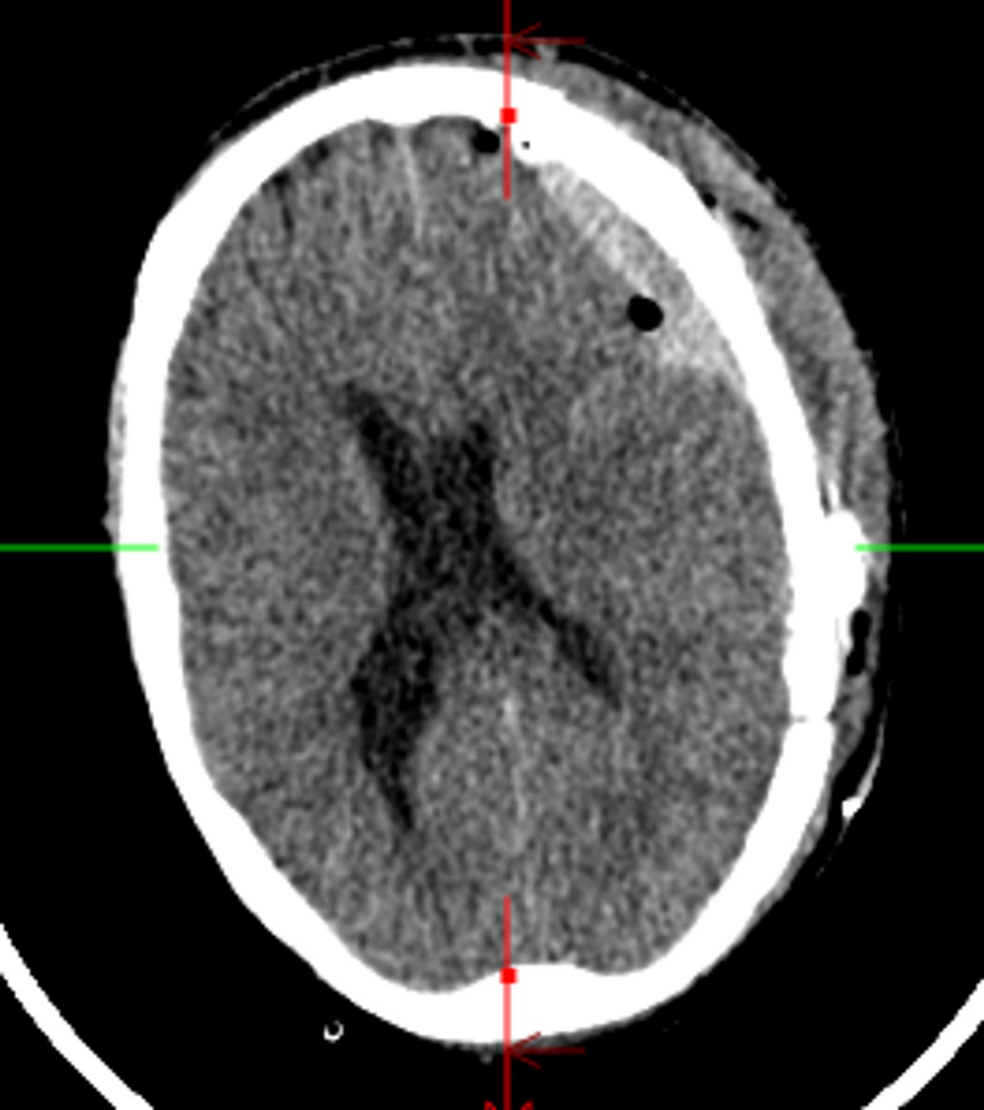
Noncontrast axial head computed tomography (CT) after decline in examination showing SDH without interval worsening. SDH = subdural hematoma
